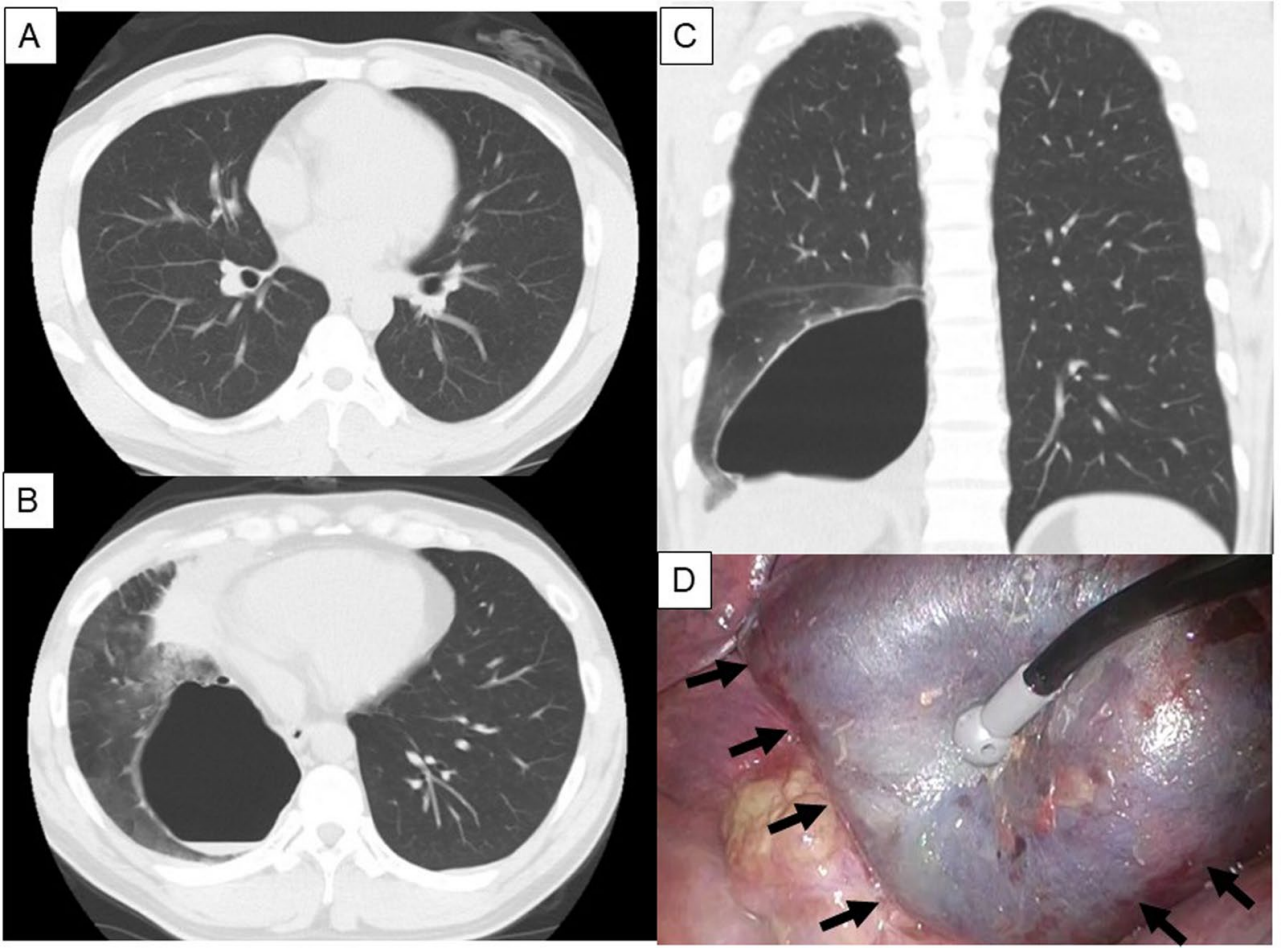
Preoperative CT and newly formed pulmonary cyst in Case 3. Preoperative CT shows normal lung (A). Postoperative CT performed 2 months after surgery reveals newly developed pulmonary cyst at diaphragmatic surface of middle lobe with some fluid inside the cyst (axial section: B, coronal section: C). Broad-based huge pulmonary cyst is recognized at re-operation (arrows) (D)
